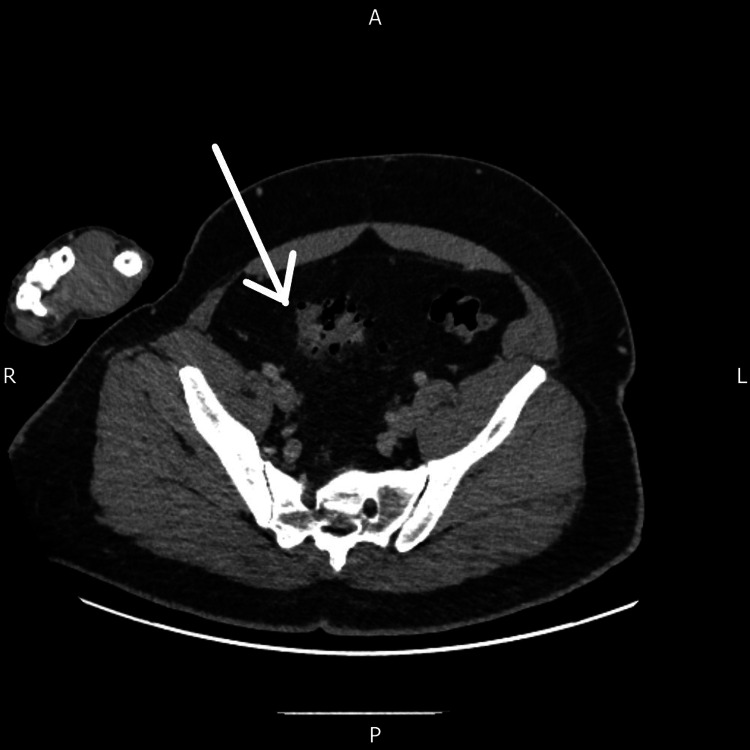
CT scan of the abdomen and pelvis demonstrating inflammatory changes in the mid sigmoid colon surrounding diverticula, suggestive of diverticulitis, seen in the axial view
